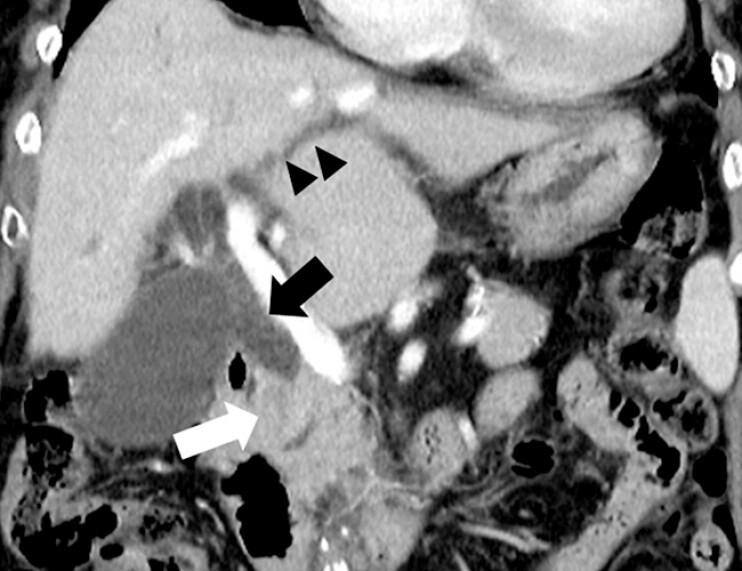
Coronal contrast-enhanced computed tomography images showing an enhancing mass (white arrow) in the distal common bile duct with upstream dilation of the intrahepatic (black arrow heads) and extrahepatic bile ducts (black arrow), suggestive of distal bile duct cancer.
